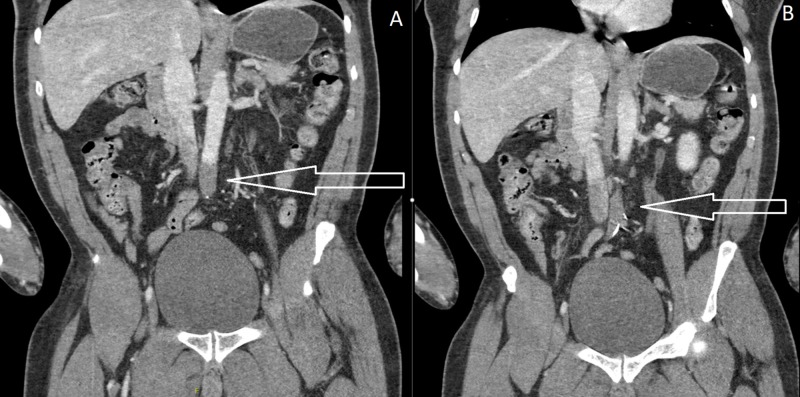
CT demonstrating distal aortic thrombus with filling defect (4A) and thrombus saddling into bilateral common iliac arteries (4B)
